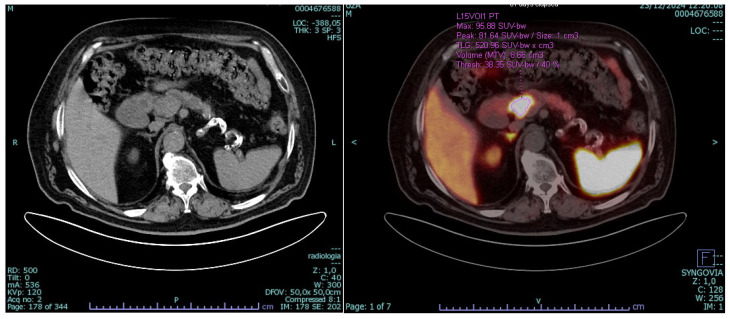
Gallium-68 PET/CT showing intense uptake (SUV max 95) in the cephalopancreatic lesion.
